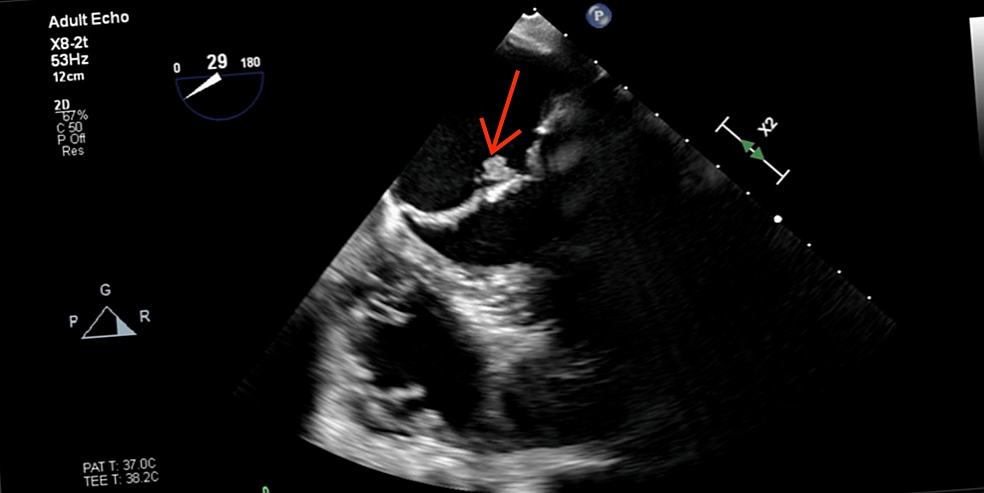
A large mobile vegetation involving the anterior mitral valve demonstrated on a repeat transesophageal echocardiogram
